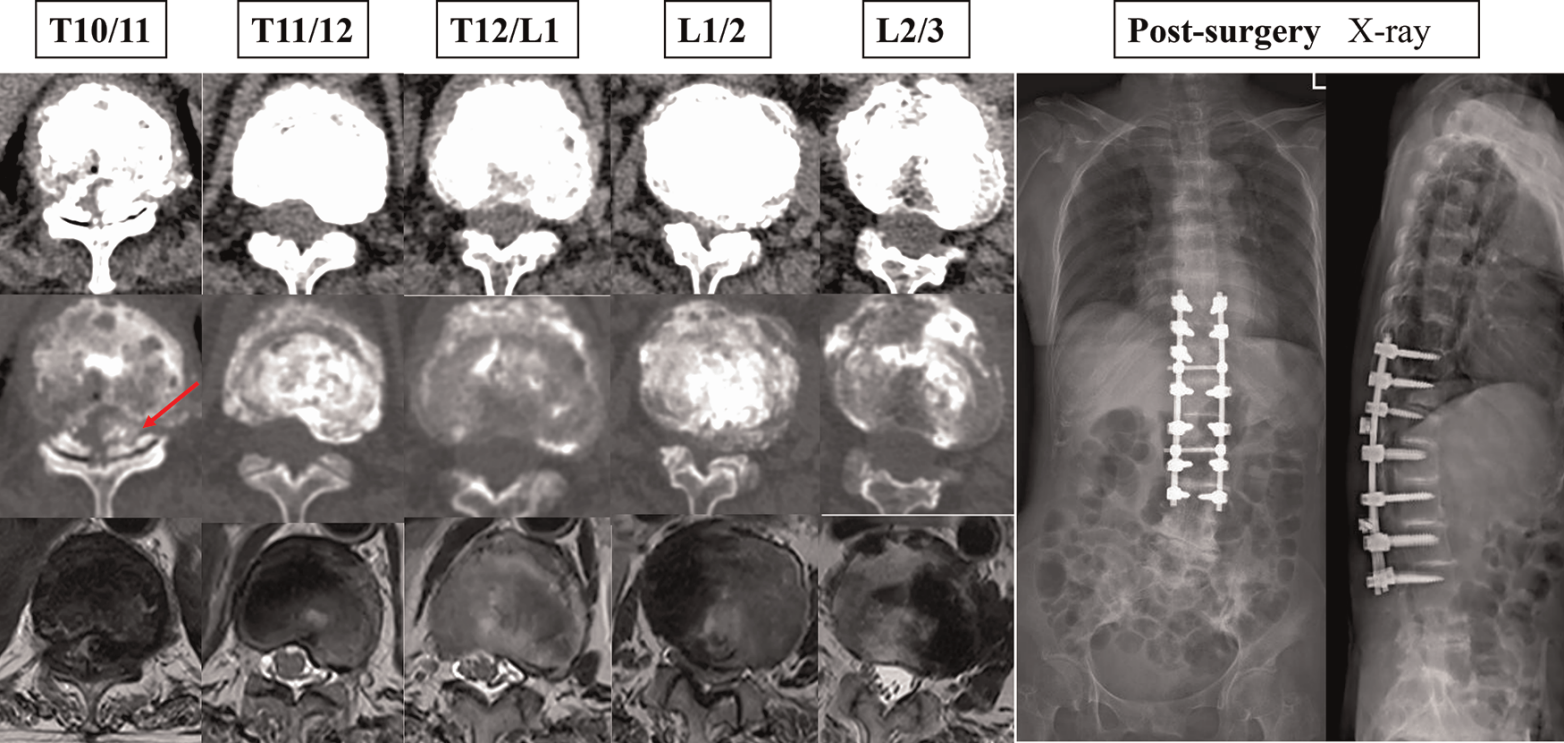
The patient's preoperative cross-sectional imaging examination and postoperative x-ray maps.
